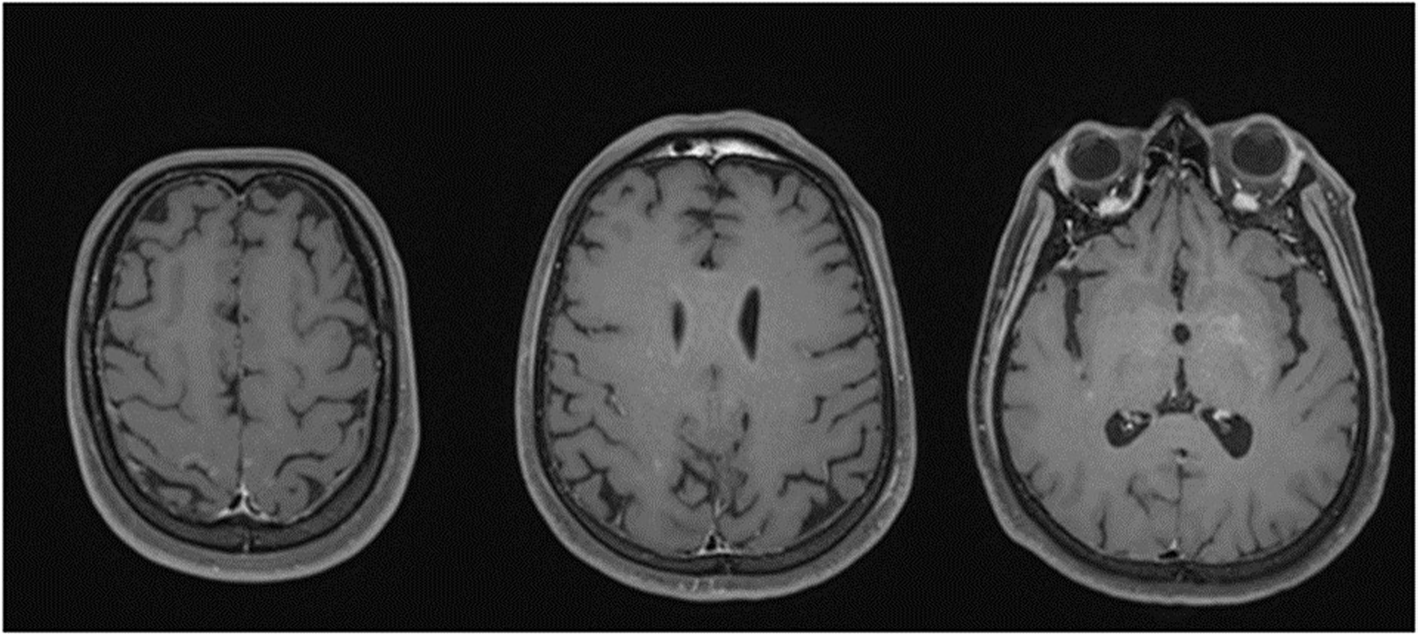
T1 contrast axial image of MRI brain shows linear enhancement along the corticospinal tracts
